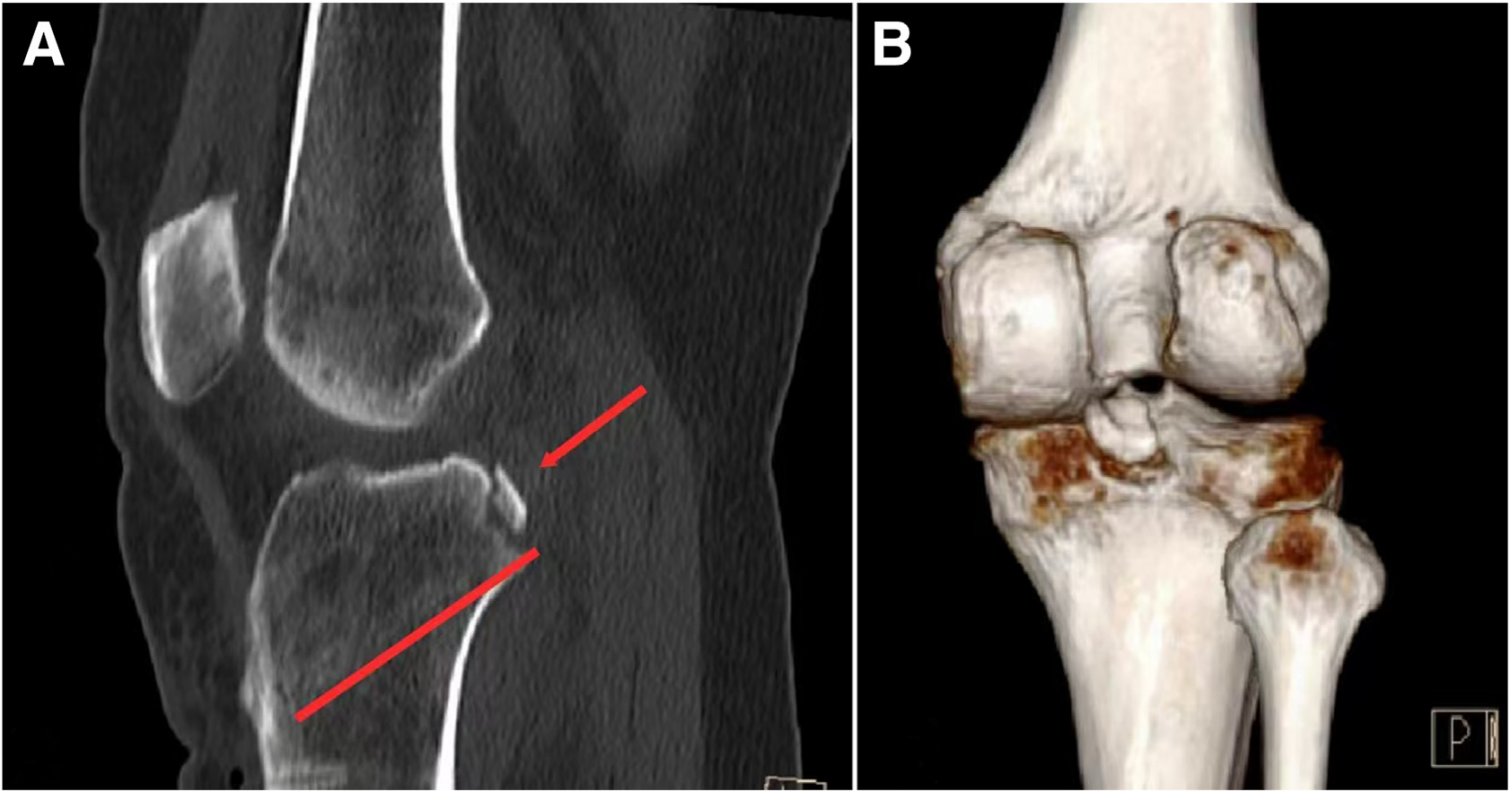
Computed tomography scan and its 3-dimensional reconstruction of the patient after surgery (patient side: right).
